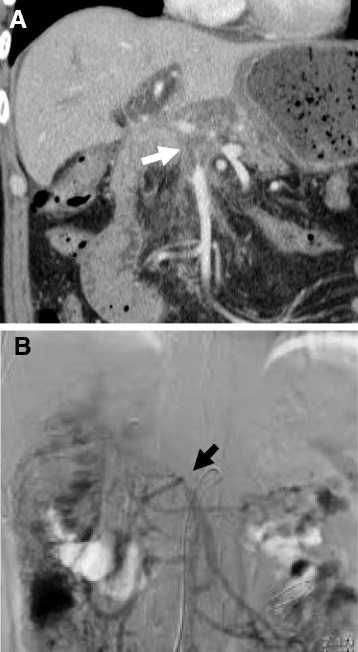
Portal phase of emergency CT (a) and angiography (b). A grossly stenotic portal vein due to postoperative pancreatic fistula was confirmed (white and black arrow)
